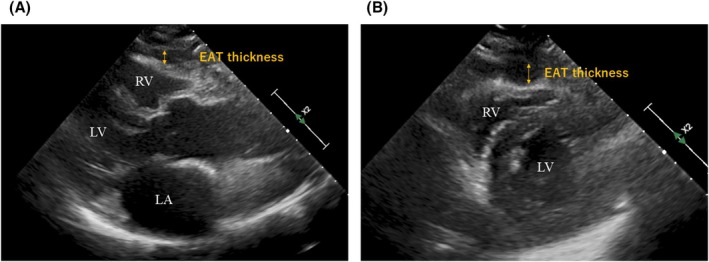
Typical examples of the echocardiographic measurement of the EAT thickness. We analyzed the preoperative echocardiograms retrospectively and measured the EAT thickness twice in the long (A) and short‐axis views (B).
